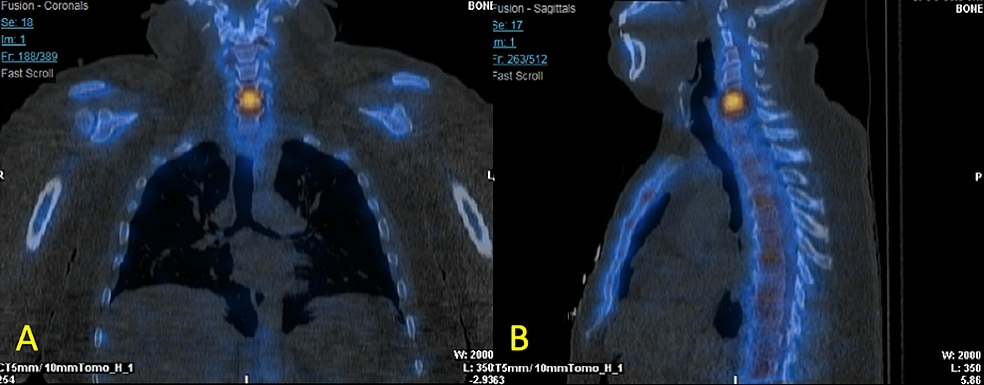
Single-photon emission computed tomography (SPECT) Coronal view (A) and sagittal view (B) SPECT demonstrated increased radiotracer uptake in the inferior half of the C5 vertebral body and the superior half of the C6 vertebral body and endplates, as well as the C5-C6 disc space, consistent with findings seen on MRI.
